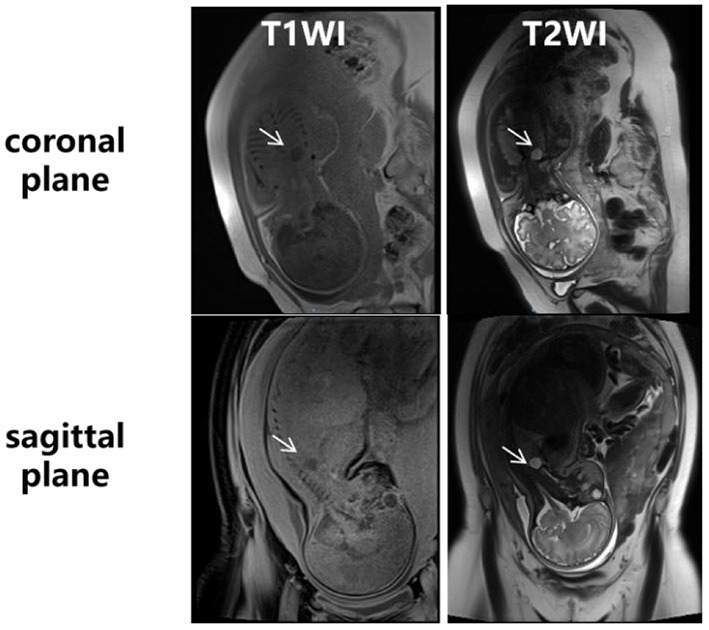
Fetal MRI at 35^+3^ weeks of gestation. An ovular-like area with long T1 and long T2 signal (white arrows) was found in the upper right thoracic cavity, on the right side of the lower trachea, above the ridge of the trachea. The boundary was clear, and the size of the lesion was about 1.5 × 1.2 × 1.5 cm.
